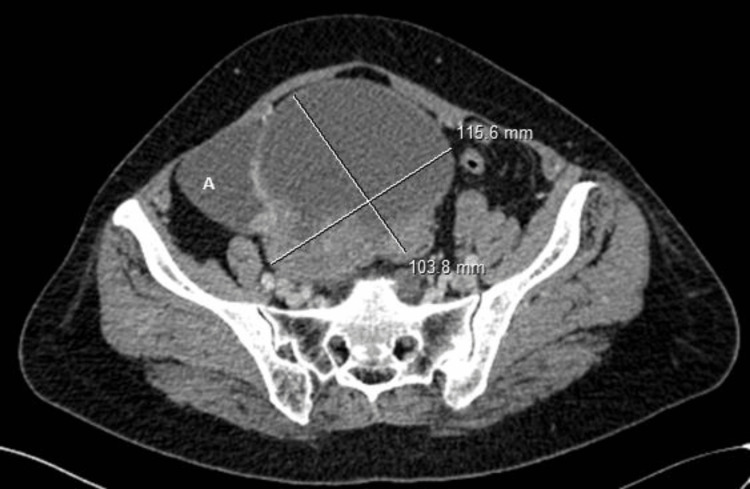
Abdominal computed tomography showing a solid-cystic pelvic mass with ascites (A) A: ascites
